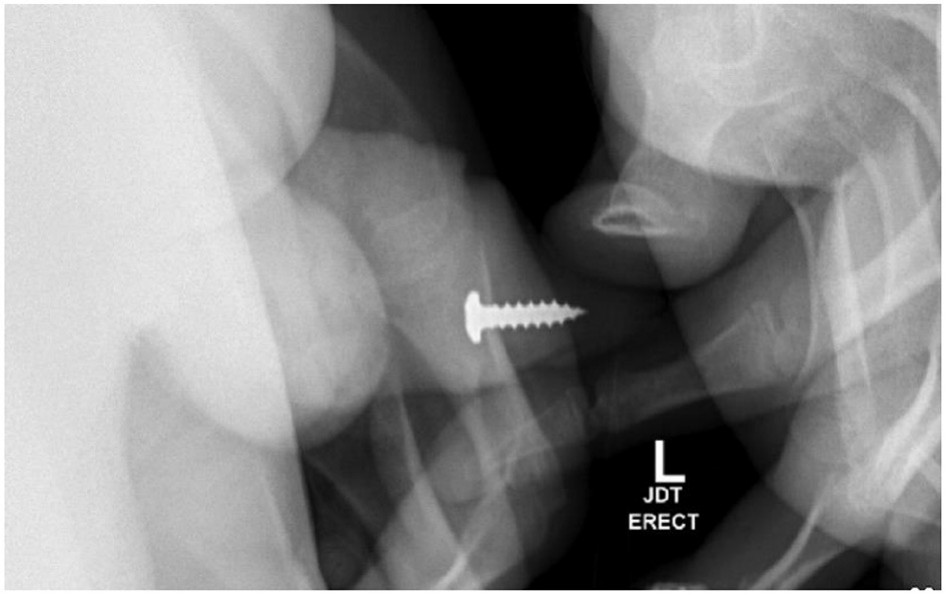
Pelvic X-ray identifies metal screw in distal penile urethra, which was removed at bedside using a hemostat.
